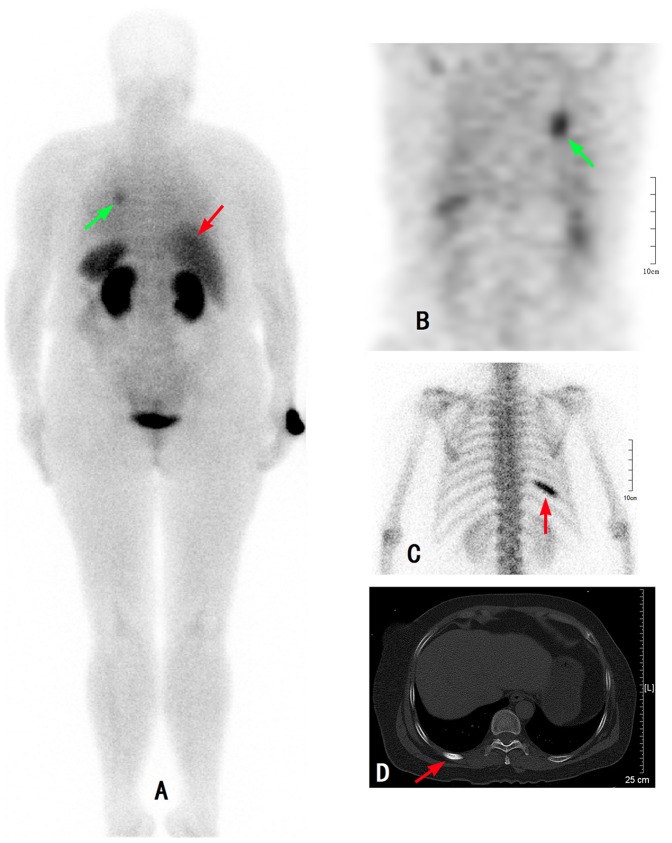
Comparison of ^99m^Tc-3PRGD2 SPECT, ^99m^Tc-MDP bone scan (posterior), and CT scan in a 62 year-old woman with left upper lung adenocarcinoma (green arrow) and costal metastasis (red arrow). A:^ 99m^Tc -3PRGD2 WB(+), B: ^99m^Tc -3PRGD2 SPECT (+), C: ^99m^Tc -MDP bone scan (+), D: CT (+). WB: whole body.
